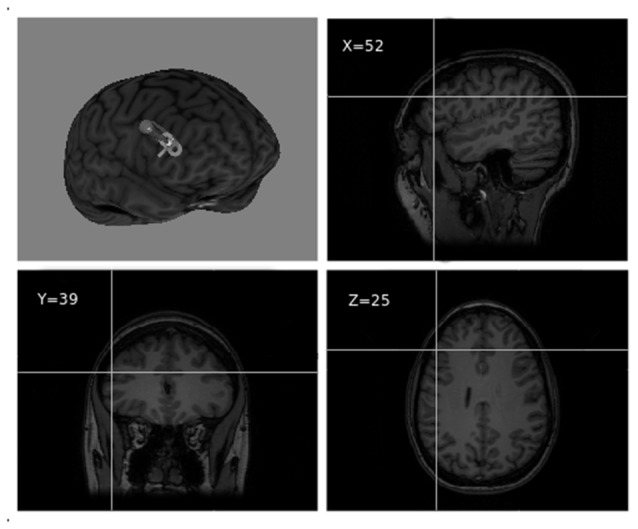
Right dorsolateral prefrontal cortex (DLPFC) TMS target visualized over a 3D MRI reconstruction of a participant’s head (reproduced with participant’s permission).
